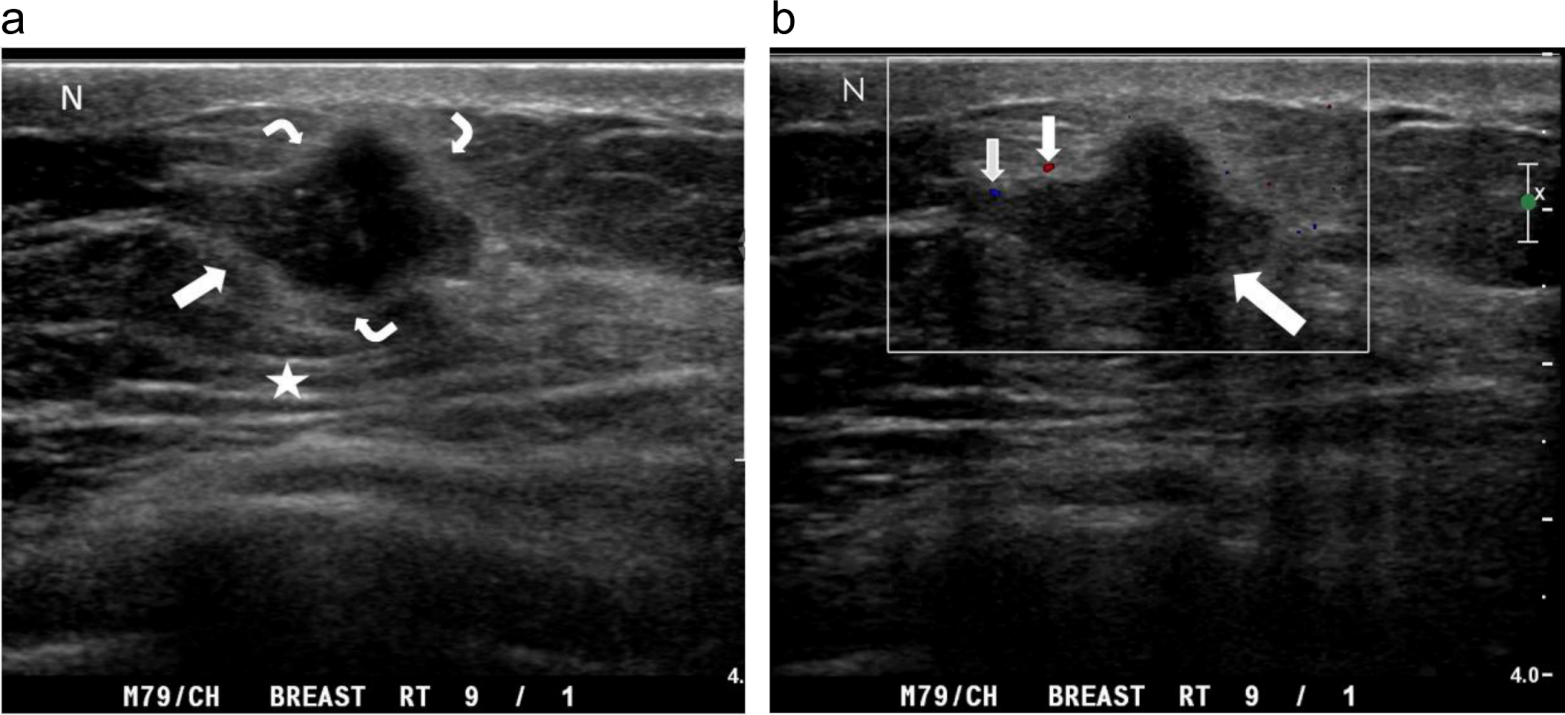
A palpable lump in the right breast of a 79-year-old man. (a) Radial ultrasound shows an irregular shape, a noncircumscribed margin, and a heterogeneously hypoechoic, subareolar, solid nodule (straight arrow). The nodule has a parallel orientation, an echogenic halo (curved arrows), posterior acoustic enhancement (star), and a markedly eccentric relationship to the nipple (N). (b) Color Doppler ultrasound shows transient occasional color pixels (short arrows) around the nodule (long arrow), classified as a low color flow signal with vessels predominantly in the rim. The postoperative pathological diagnosis is invasive ductal carcinoma.
